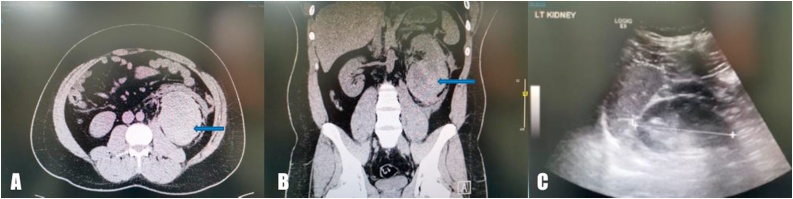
A, B: Follow up CT scan showed reduction of the hematoma by 50 percent compared to the initial CT scan, and there were no other abnormalities or lesions. C: Follow up renal Ultra sound showed no masses, stones or hydronephrosis with normal corticomedullary differentiation.
